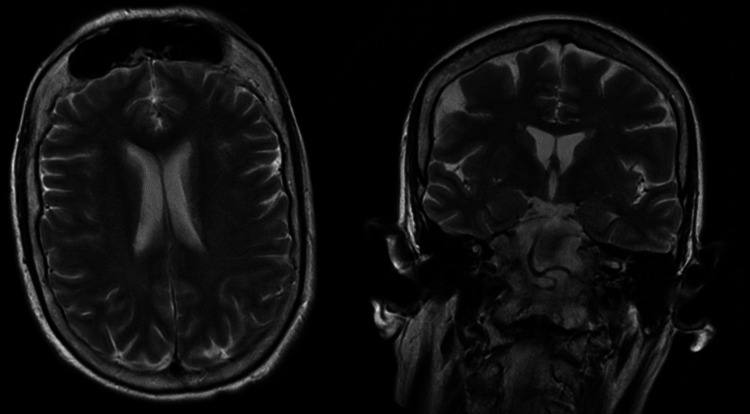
MRI of the head was unremarkable.
